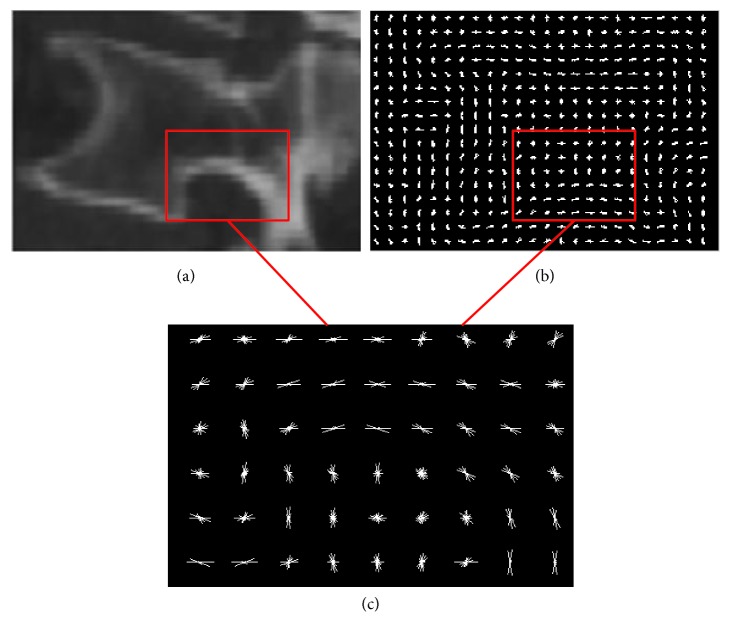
The result of the HOG descriptor: (a) CT vertebral image; (b) the visualization of HOG; (c) local amplification result.
